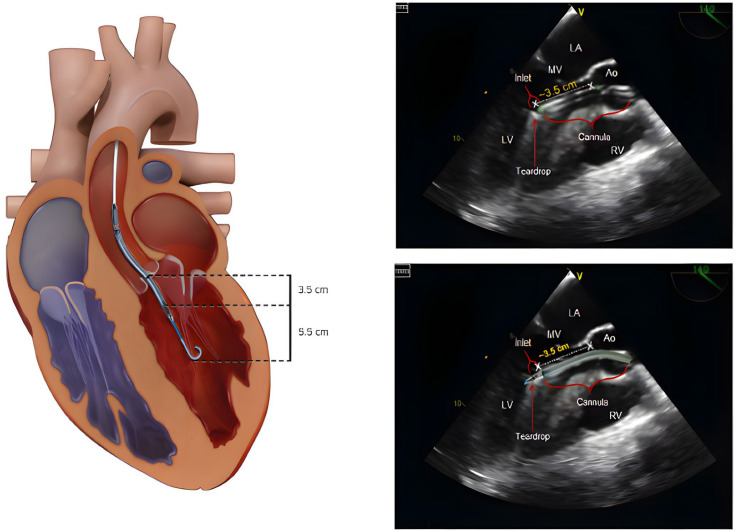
Left ventricle Impella positioning through the Aortic Outflow tract (red chamber). Left Atrium (LA), Mitral Valve (MV), Left Ventricle (LV), Aortic Outflow Tract (Ao), and Right Ventricle (RV) are visualized in the echocardiogram images. The 3.5 cm and 5.5 cm measurements are echocardiographic depth landmarks confirming correct Impella positioning, with the inlet below the aortic valve in the LV and the outlet in the ascending aorta. Open-access image obtained from Zein et al. A Review of the Impella Devices [36].
